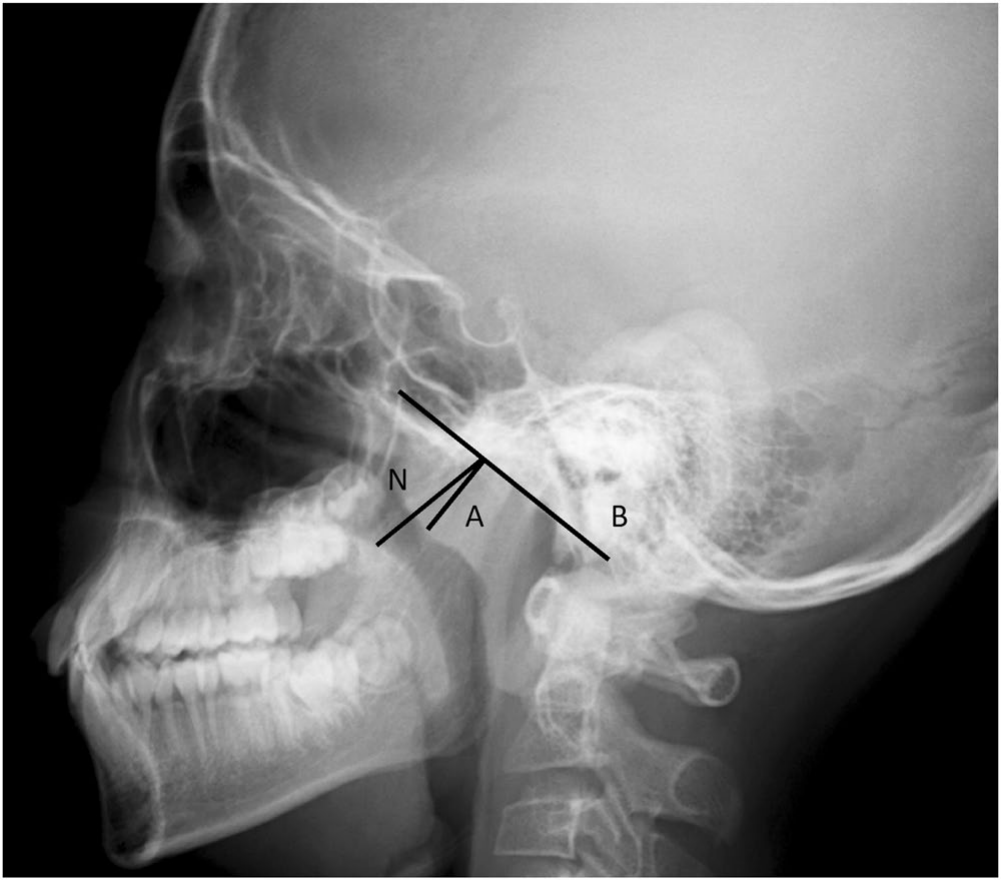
Measurement of the adenoidal/nasopharyngeal (A/N) ratio on lateral cephalometric radiographs. The distance A is measured along the line running perpendicular from the point of maximal convexity along the inferior margin of the adenoidal shadow to the point of intersection with line B. The nasopharyngeal space N is the distance between the posterior/superior edge of the hard palate and the anterior/inferior edge of the sphenobasioccipital synchondrosis. The A/N ratio is obtained by dividing A by N.
